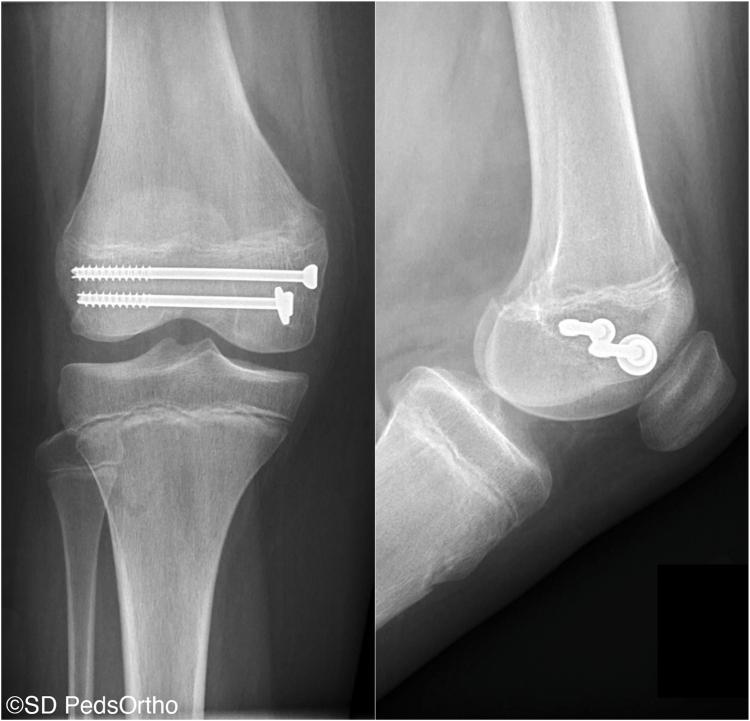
Radiograph taken three months postoperatively. Her distal femoral physis appears somewhat narrowed, and she will be closely followed to determine if she develops angular deformity or leg length discrepancy.
